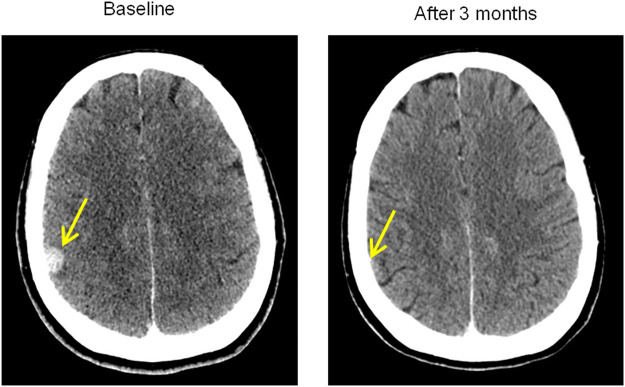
Brain response at CT scan. Comparison between baseline brain lesion at diagnosis and the impressive complete response after 3 months following chemoimmunotherapy and osimertinib. Osimertinib was only taken for a total of 37 days and then suspended because of severe toxicity.
